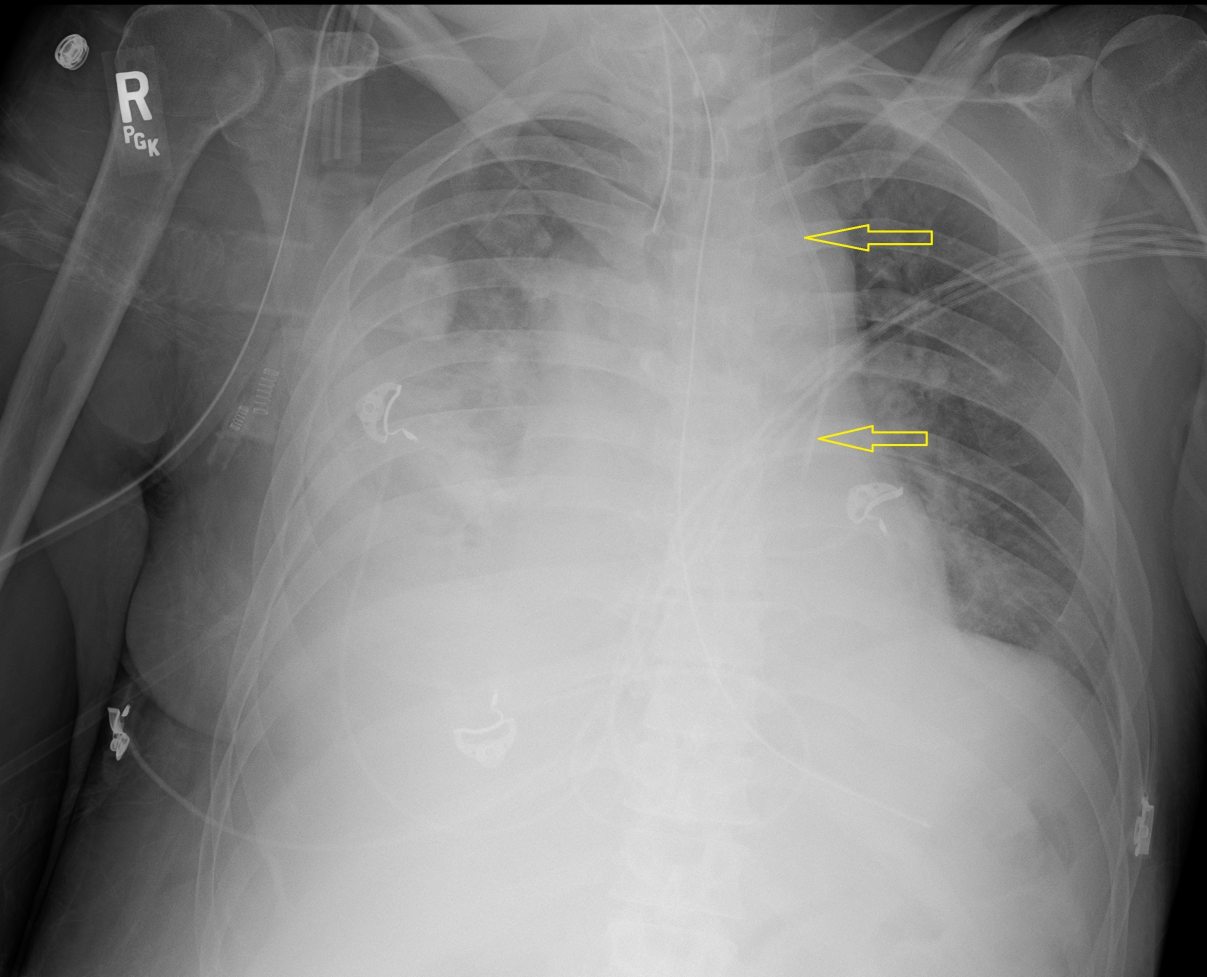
Chest x-ray confirming the placement of the central venous access line. The line (marked by yellow arrows) does not take the expected course toward the right side of the heart.
